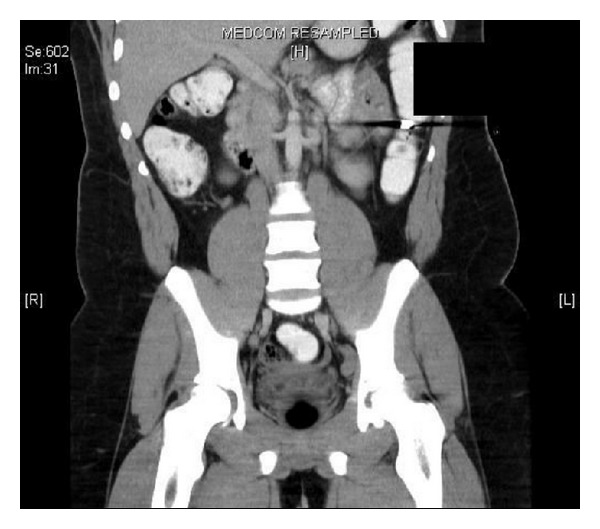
A CT reveals a bullet in the lumen of the small intestine in the right upper quadrant.
